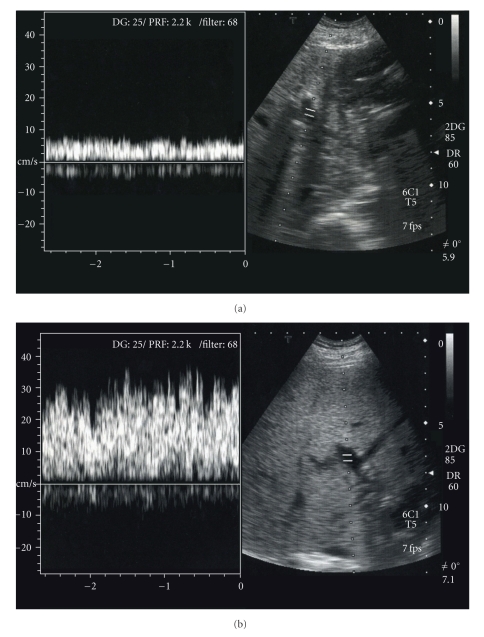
Doppler ultrasonography shows gradual decrease of the portal vein peak velocity of the left portal branch and inverse increase of the portal vein peak velocity of the right portal branch.
